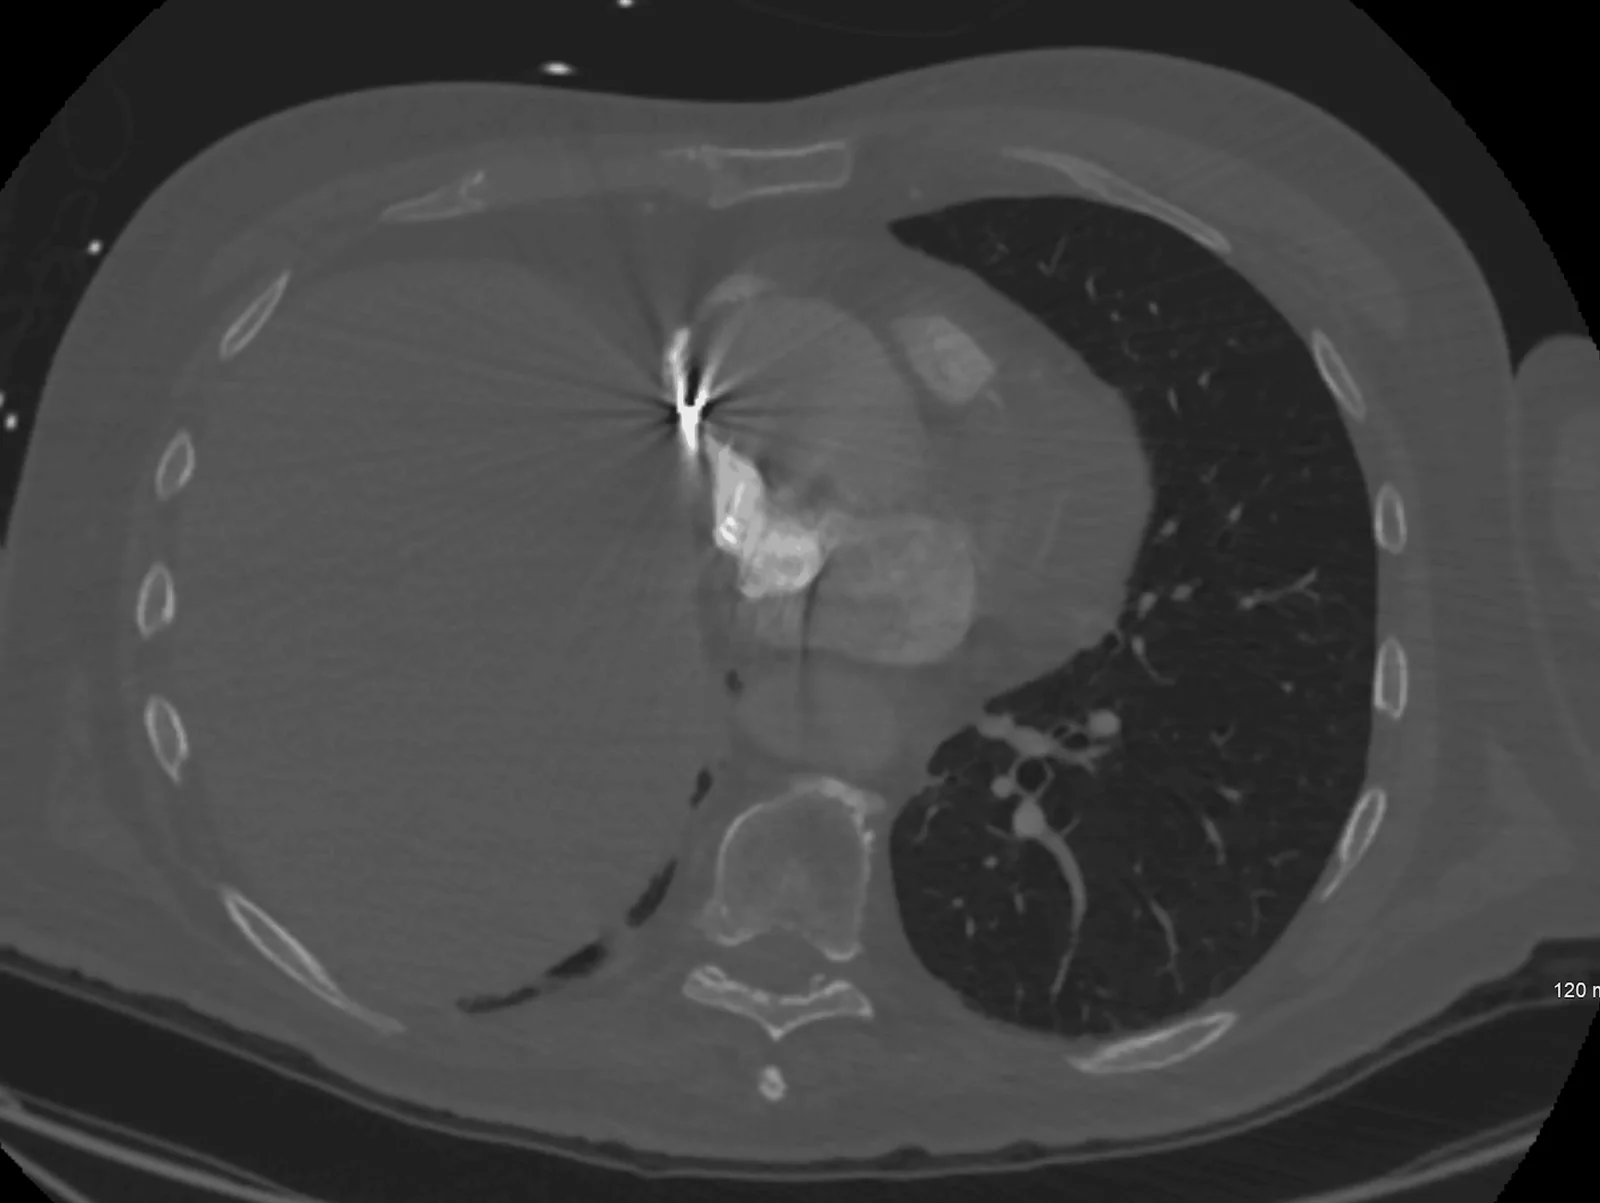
Contrast-enhanced CT pulmonary angiographic image at the level of the atria demonstrating a contrast jet from the right atrium to the left atrium, consistent with right-to-left interatrial shunting
